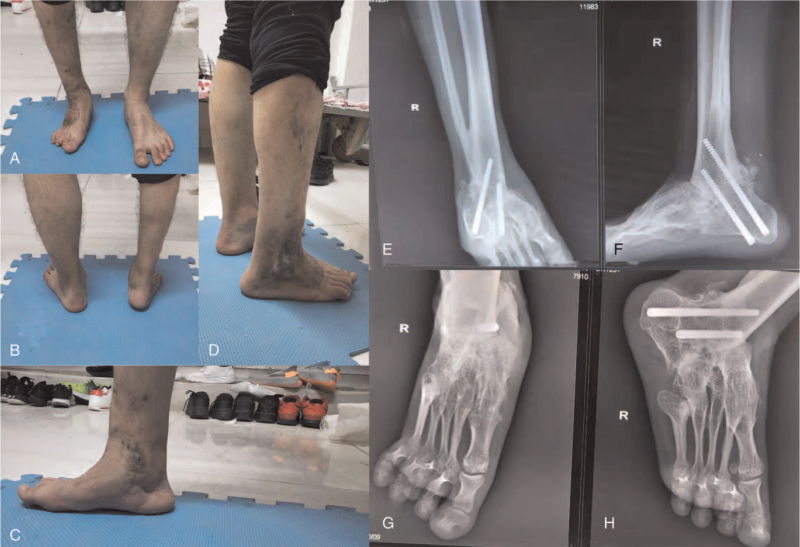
One year follow-up appearance and X-ray images. (A, B) The valgus of the hindfoot and the supination of the forefoot were corrected. (C) The arch of the foot was restored. (D) The cicatricial tissues of the lateral malleolus and the dorsum pedis were safely preserved. (E–H) All osteotomy sites got bony union.
